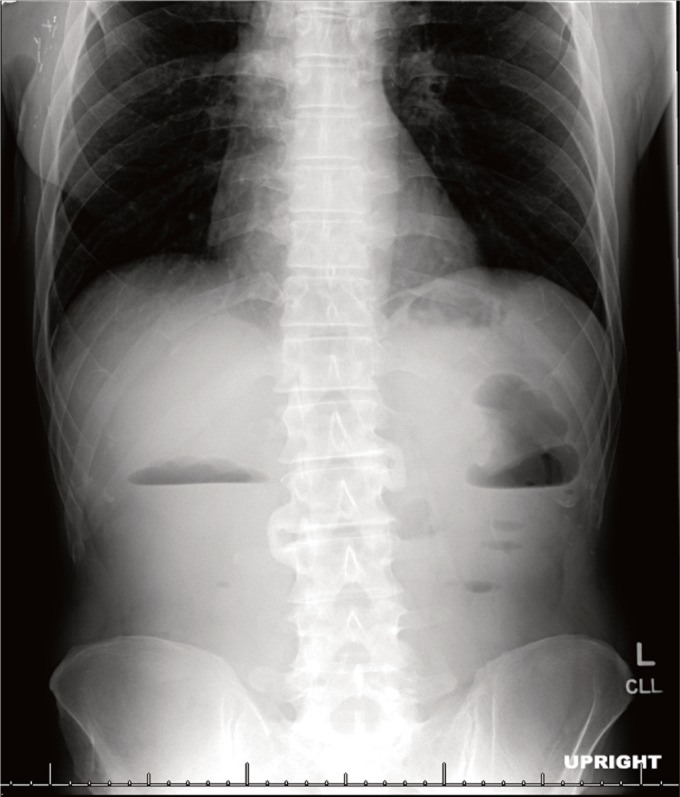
Abdominal x-ray on presentation revealing air-fluid levels.
